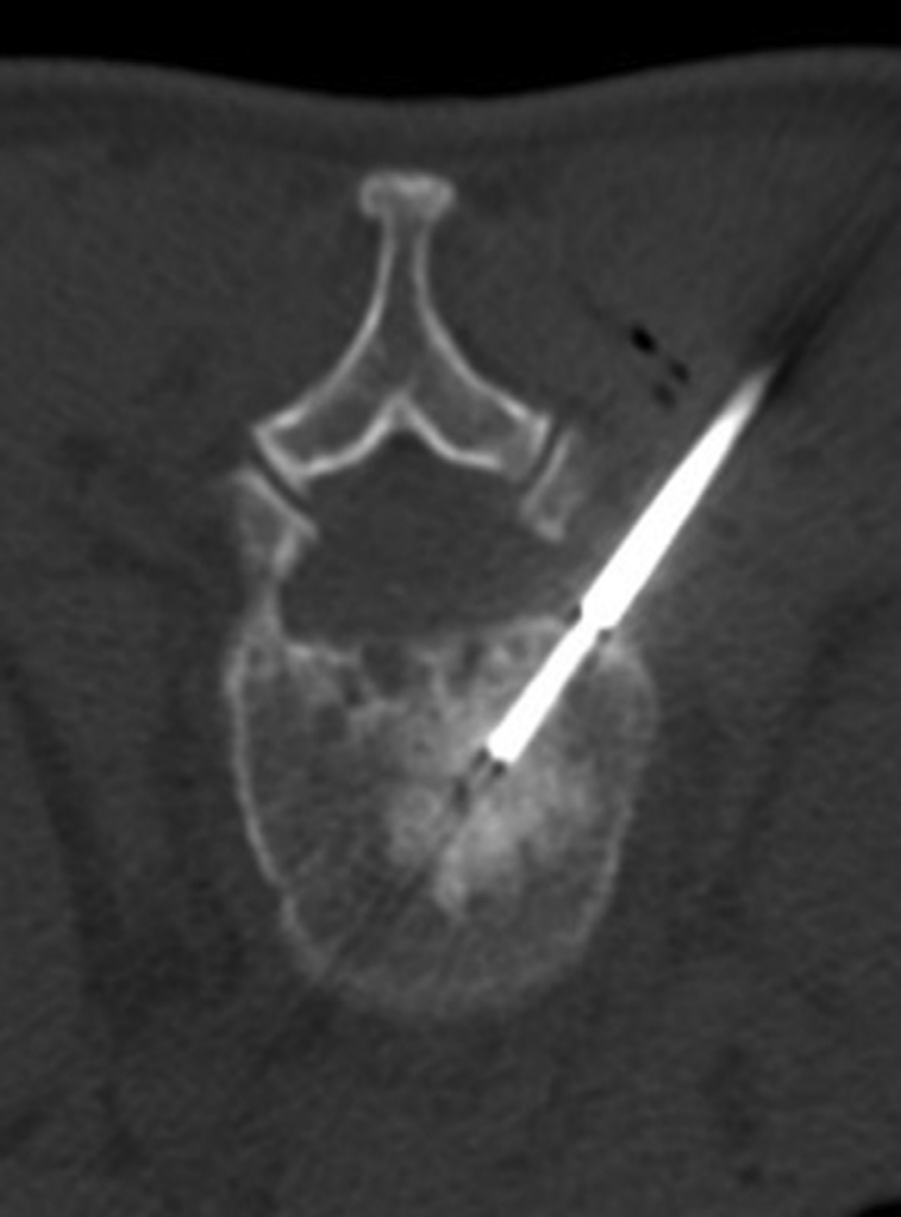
Representative image showing CT-guided biopsy of the L2 vertebral body sclerotic lesion from a right posterolateral approach.
